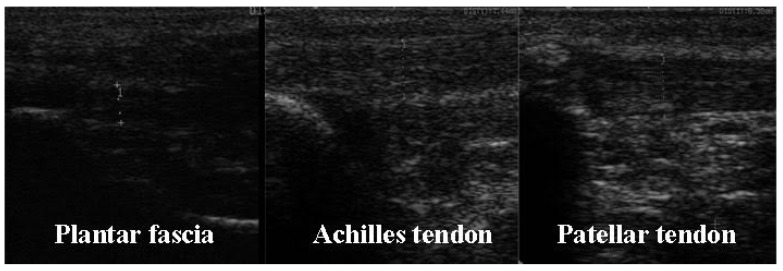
Images of the ultrasound scanning in the Plantar fascia, Achilles tendon, and Patellar tendon.
